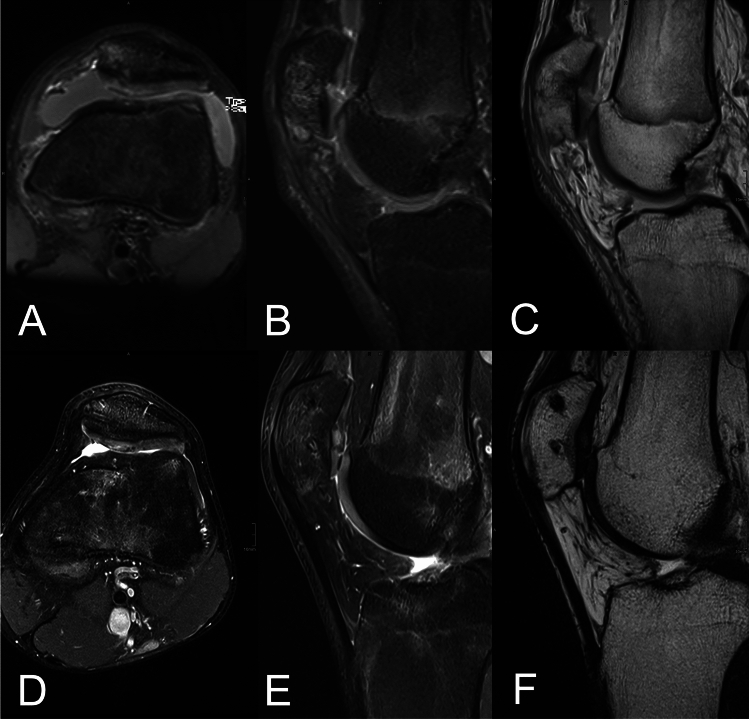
Typical magnetic resonance imaging scans of a 19-year-old male patient with a retropatellar cartilage defect ICRS grade IV with subchondral reaction and joint effusion: A axial PD-weighted with fat saturation, B sagittal PD-weighted with fat saturation, and C sagittal PD-weighted sequences. MRI scans obtained 5 years after MACI with Novocart® 3D and concomitant medial patellofemoral ligament reconstruction showing complete filling of the defect with complete integration, intact surface of the repair tissue, and an inhomogeneous structure. The subchondral lamina and bone are intact, with no adhesions or effusion: D axial PD-weighted with fat saturation, E sagittal PD-weighted with fat saturation, F and sagittal T2-weighted sequences
